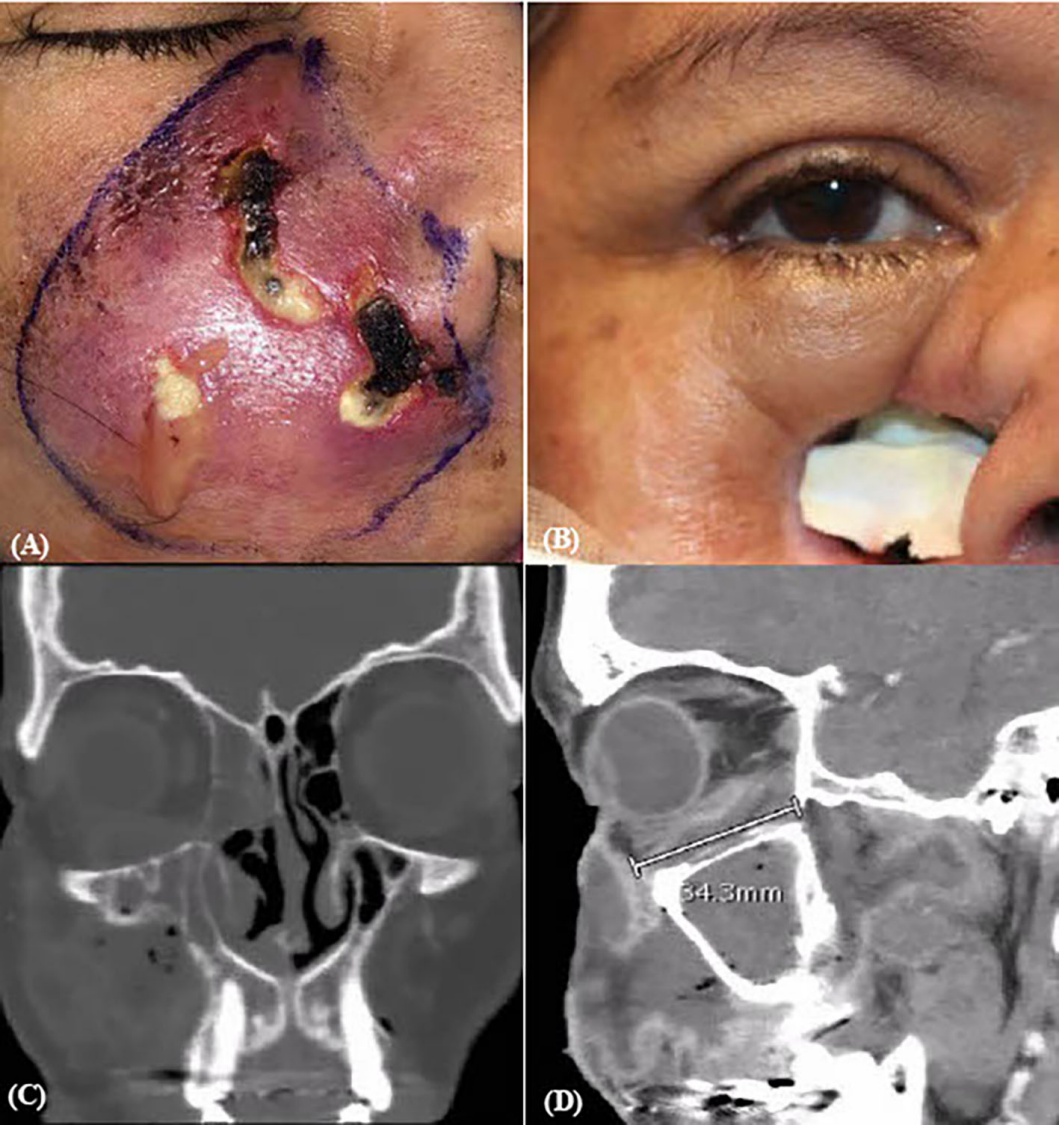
Pre- and post-operative surgical and CT imaging of case 1. (A) Pre-op ESS and (B) two months post-discharge gross images. CT orbit coronal (C) and sagittal (D) sections revealing continued mucormycosis extension into middle cranial fossa and adjacent structures.
